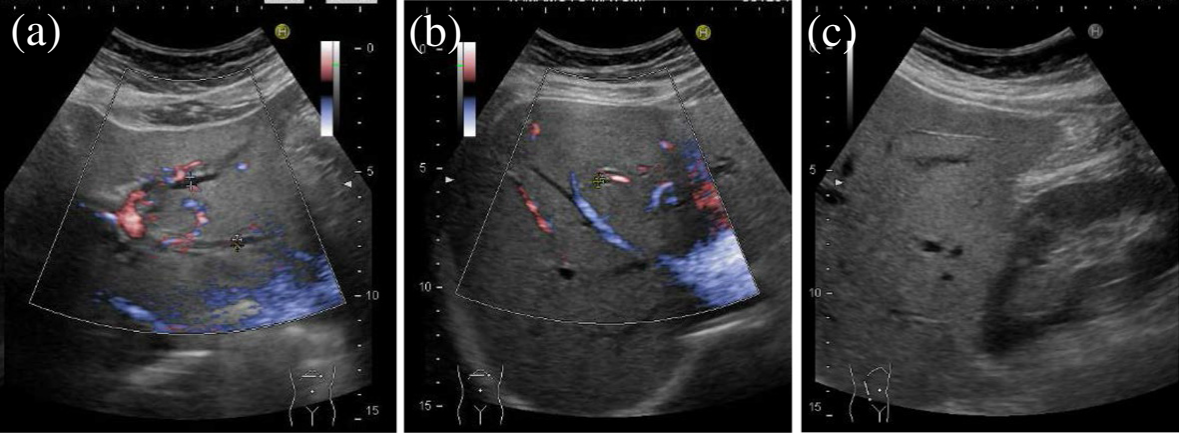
Abdominal ultrasound examination reveals the intrahepatic bile ducts in the left lobe expanded up to 3.3 mm (a) and slight enlargement of the intrahepatic duct in the right lobe to 1.7 mm (b). Hepato-renal contrast is observed, which suggested the possibility of fatty liver (c)
